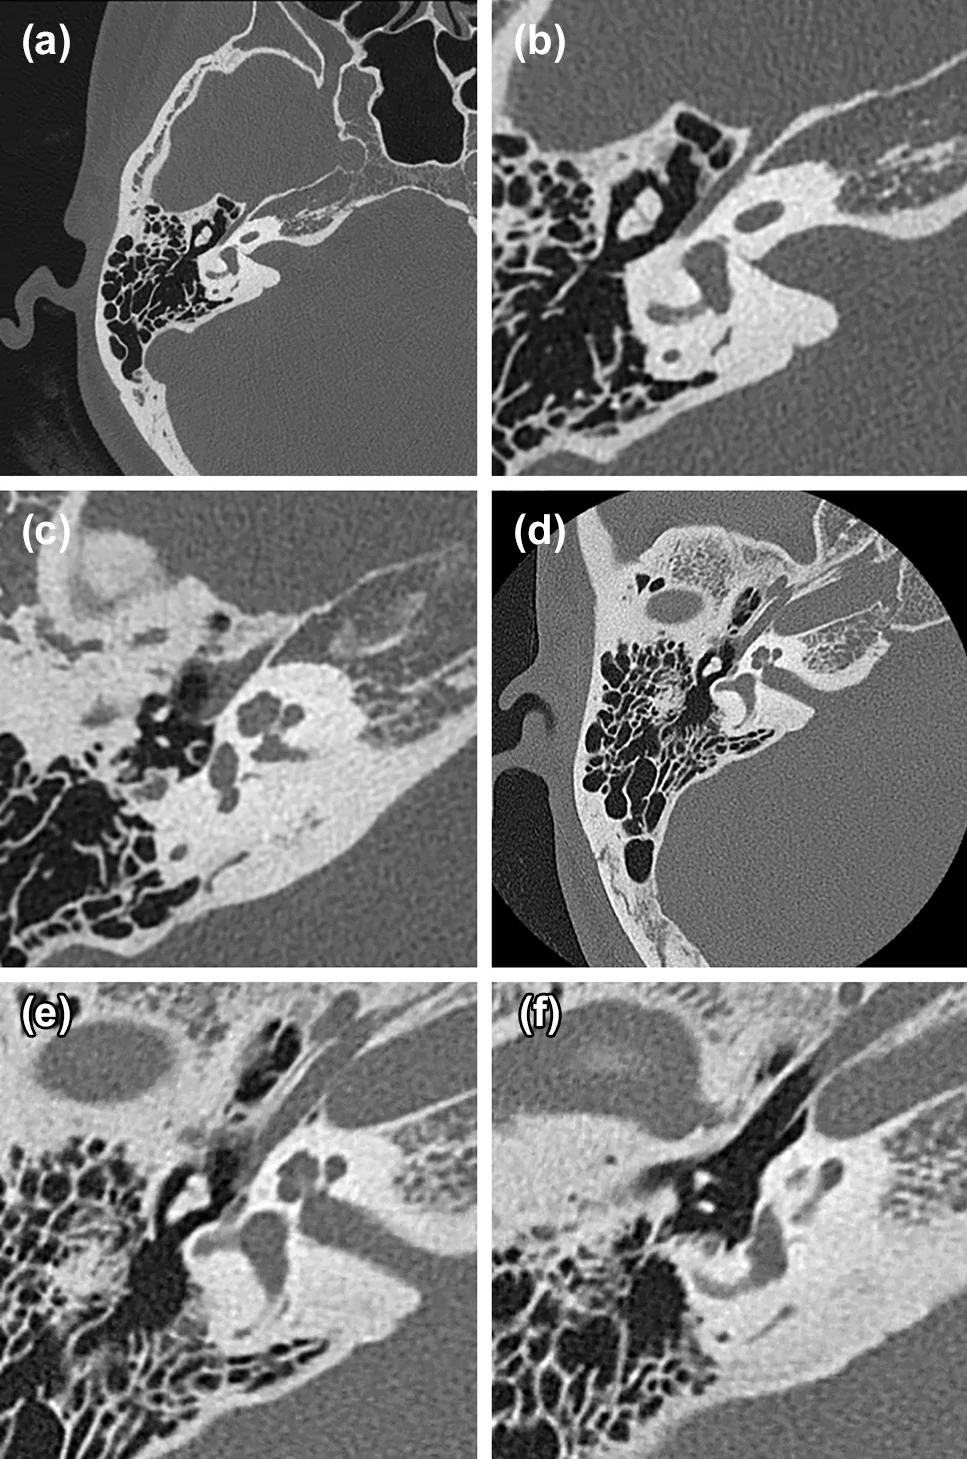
PCD-CT axial images of the right temporal bone CT at 50% doses (a–c). The visual scores for the incudomalleolar joint (b) and stapes (c) by two reviewers were both 4. EID-CT axial images of the right temporal bone CT at standard dose (d–f). The visual scores for the incudomalleolar joint (e) and stapes (f) by two reviewers were 2 and 3, respectively PCD-CT, photon-counting detector computed tomography; EID-CT, energy-integrating detector computed tomography
